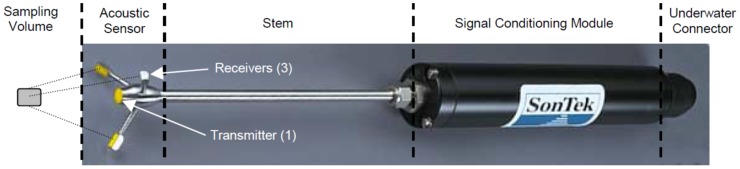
Three-dimensional Sontek Micro acoustic Doppler velocimeter (ADV) 16 megaHertz (MHz).
